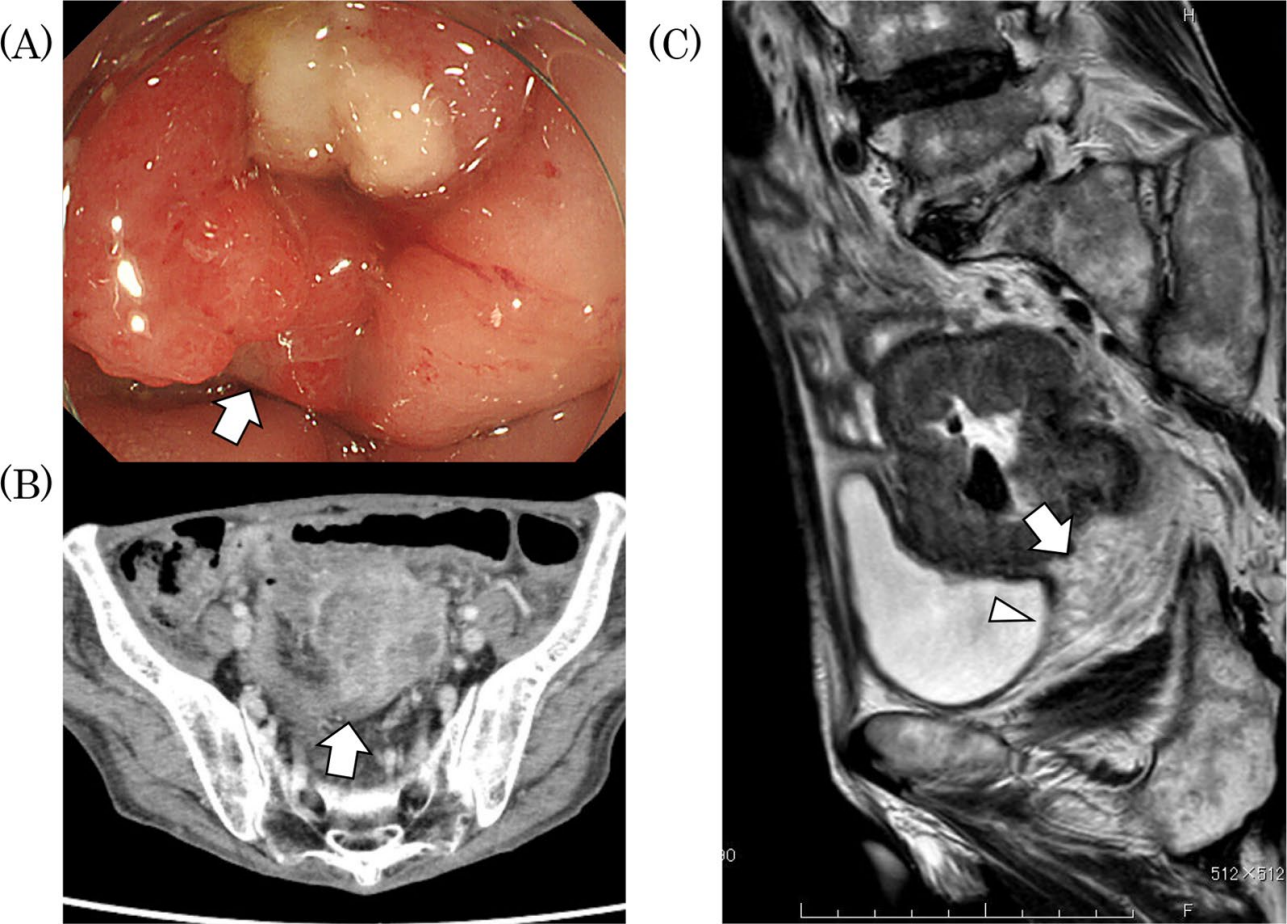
Baseline imaging for Case 2. A Endoscopic imaging showing a sigmoid colon tumor. B Computerized tomography (CT) scan staging a locally advanced sigmoid colon cancer (cT4bN1bM0). C Magnetic resonance imaging (MRI) showing a suspected tumoral invasion to the bladder (arrowhead). Arrows identify the tumor
